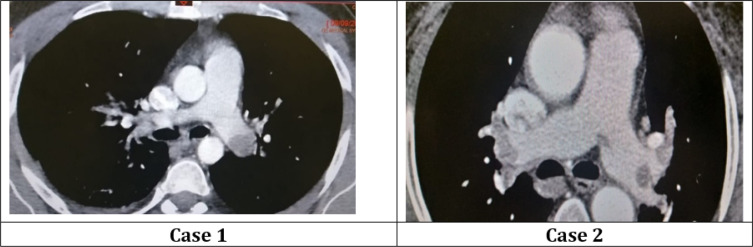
Axial cut of thoracic computed tomography angiography showing embolism in right and left pulmonary artery
